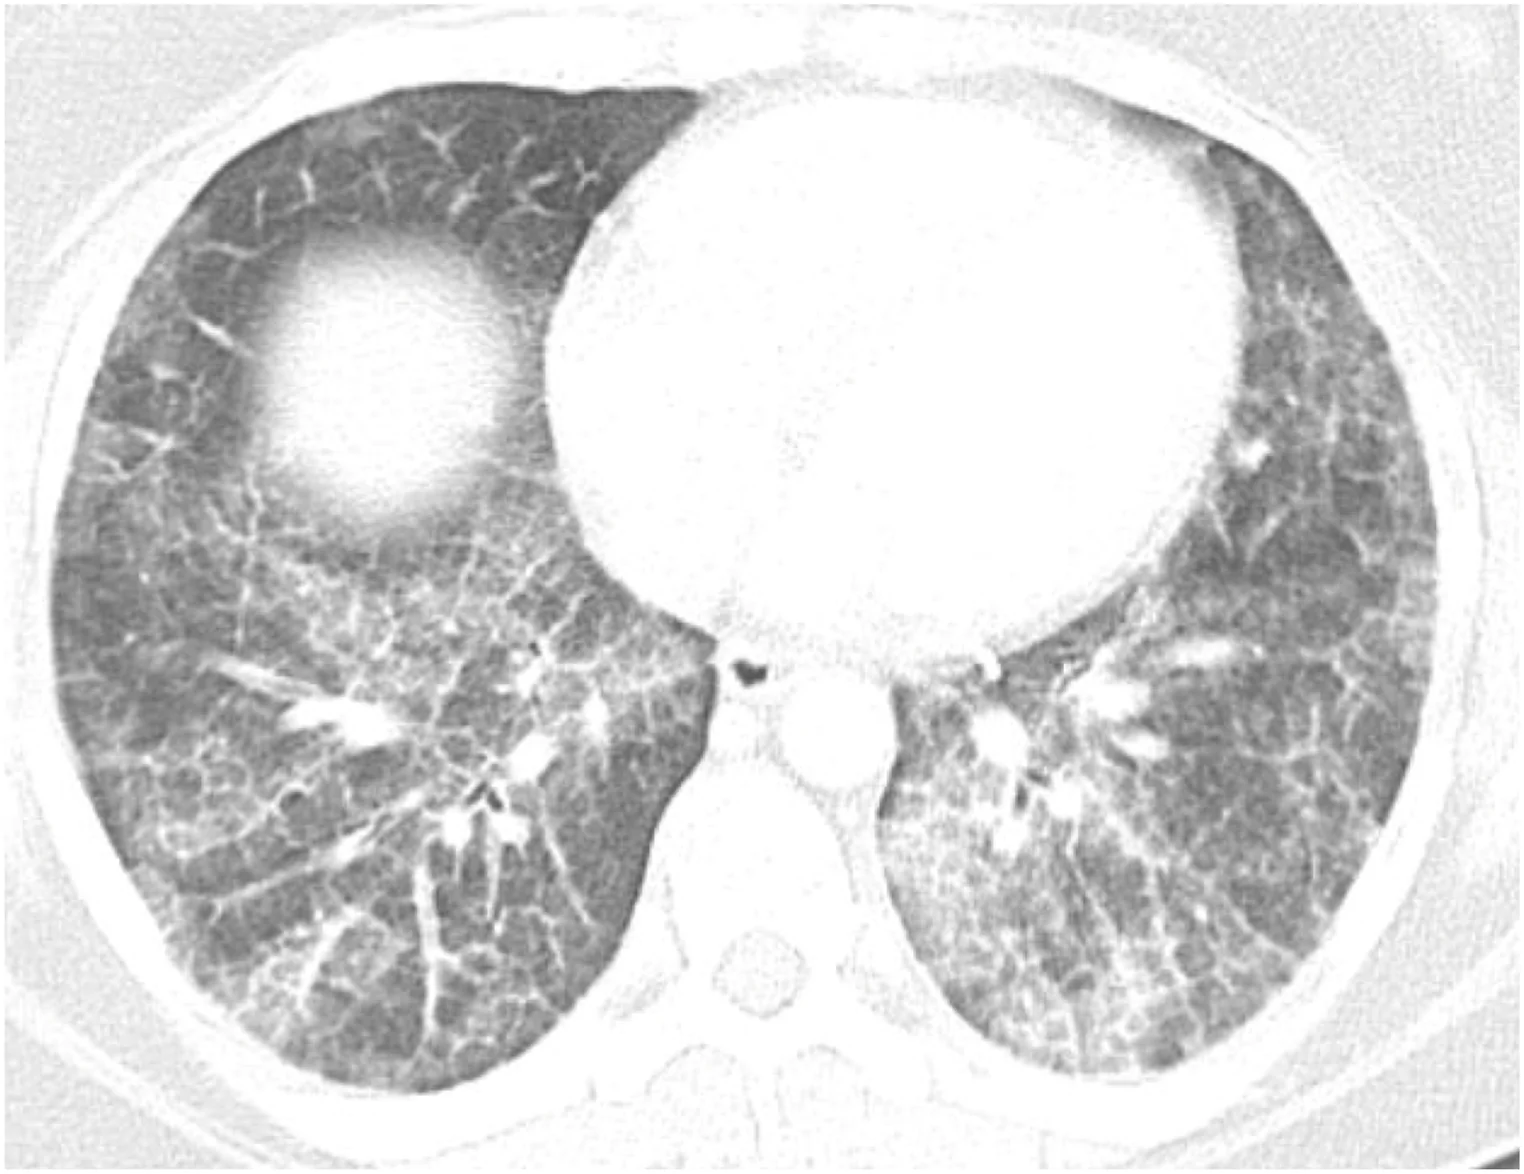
CT Chest with Contrast on initial admission demonstrating multifocal opacities and crazy paving pattern.
